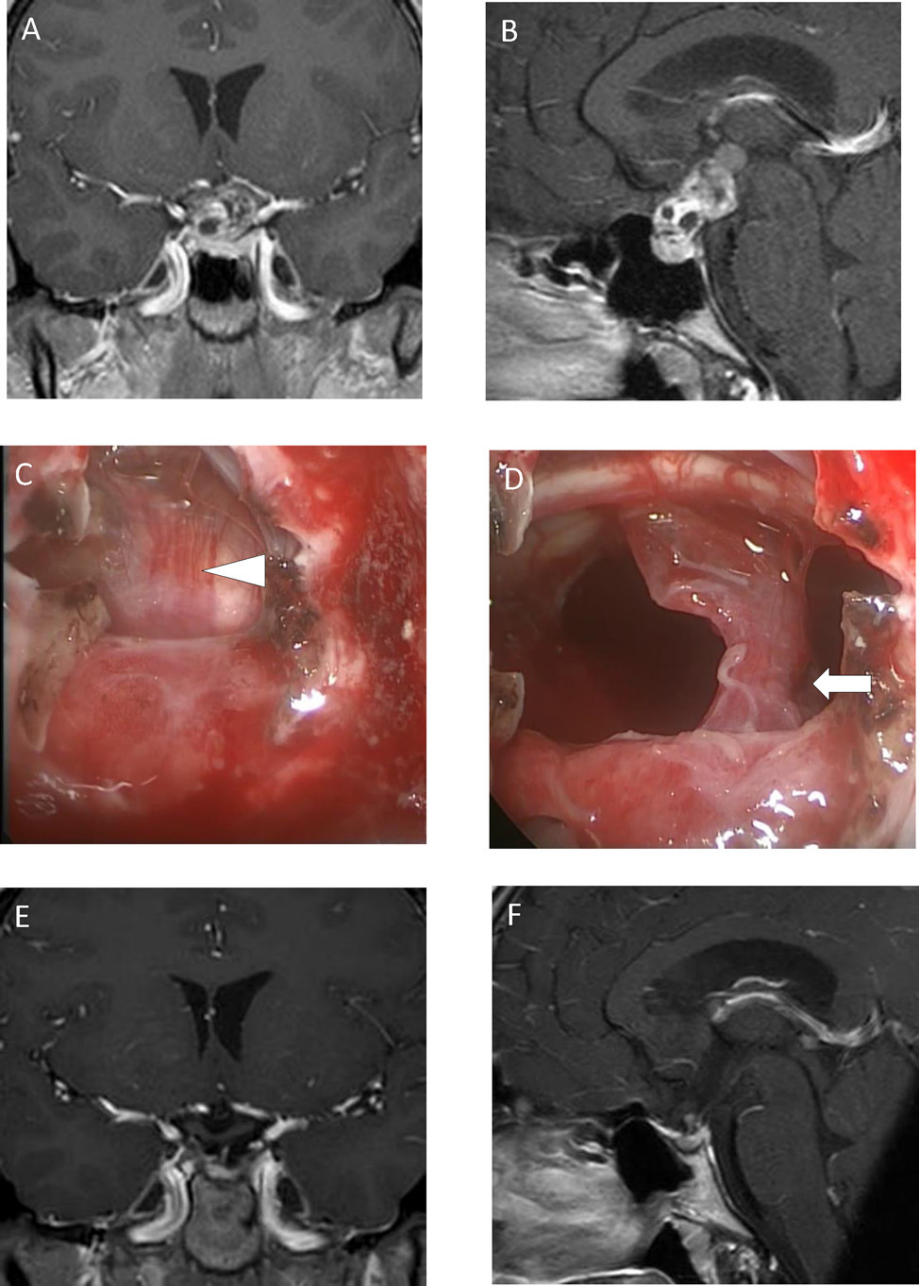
Case 3: A 14-year-old girl. Preoperative coronal (A) and sagittal (B) enhanced T1-weighted magnetic resonance imaging demonstrated a solid suprasellar tumor. The intraoperative view showed that the pituitary stalk was located rostral to the tumor (C). The arrow head points to the pituitary stalk. The tumor was removed except for the pituitary stalk and the tumor caudal to it (D). The arrow points to the residual tumor caudal to the pituitary stalk. After Gamma Knife radiosurgery, the tumor was completely controlled (E, F).
